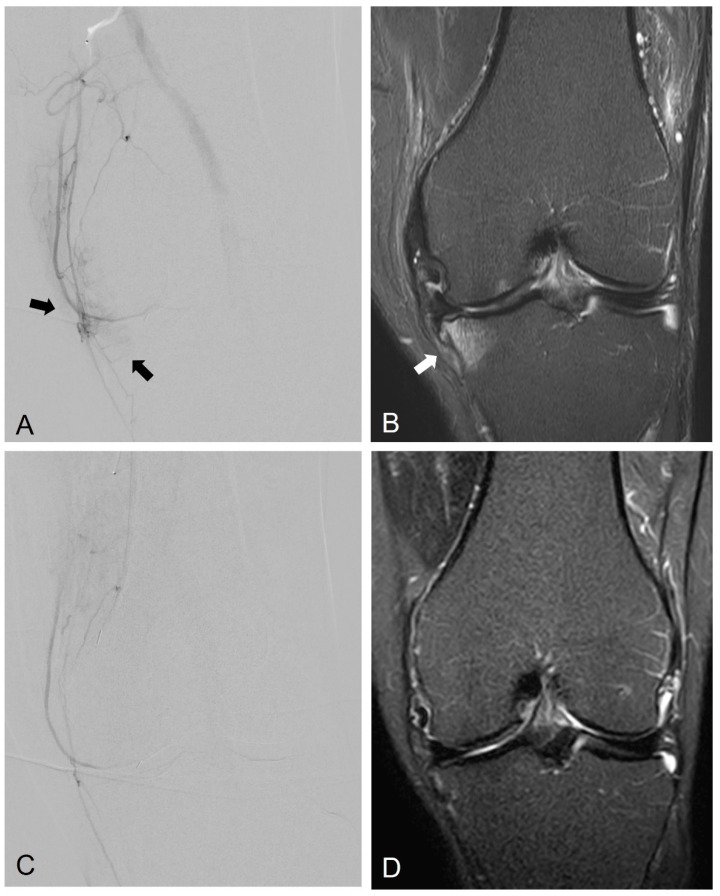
Representative pre- and postprocedural images of the right knee in a 69-year-old male with KL grade 2 osteoarthritis with associated pain. (A) Preprocedural angiography of the right descending genicular artery showed angiogenesis over medial aspect of the knee joint (black arrows). (B) Preprocedural coronal MRI (fat-saturated T2-weighted TSE) revealed subchondral bone marrow lesion over right tibia plateau (white arrow). (C) Postembolization angiography indicated elimination of angiogenesis after GAE. (D) Follow-up MRI acquired 3 months after GAE revealed the reduction of subchondral BML. The patient’s knee pain and function were improved after GAE.
